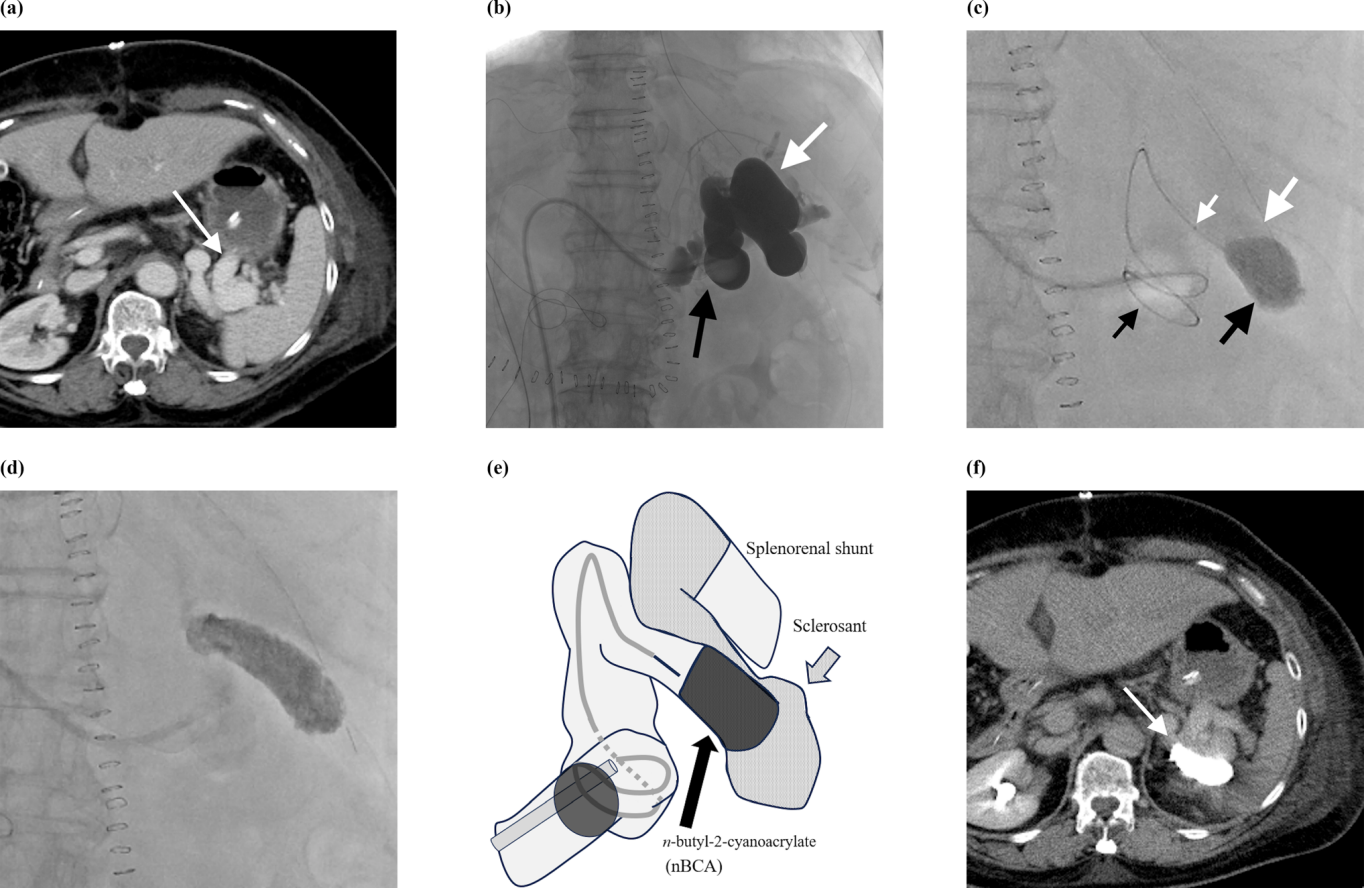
A 64-year-old female with a splenorenal shunt after liver transplantation (patient no. 4). a Contrast-enhanced CT image shows a splenorenal shunt (arrow), which caused a decrease in the portal flow to the liver graft. b After inserting a balloon catheter (black arrow) into the shunt via a right femoral vein approach, balloon-occluded retrograde venography revealed the whole shunt and stagnation of blood flow in the shunt (white arrow). c After 5% ethanolamine oleate iopamidol injection (large white arrow), 33% *n*-butyl-2-cyanoacrylate (nBCA; large black arrow) was injected with a triaxial microcatheter system (a 1.9Fr microcatheter and a 2.7Fr microcatheter; small white arrow) under balloon flow control (small black arrow). d The injected nBCA formed a large cluster in the shunt and was stable. e The scheme shows n-butyl cyanoacrylate-associated retrograde transvenous obliteration for a splenorenal shunt with a triaxial system under balloon flow control. f Follow-up contrast-enhanced CT performed 66 days after embolization shows the nBCA cluster in the shunt (arrow), consistent with the angiographic findings, without nBCA migration
